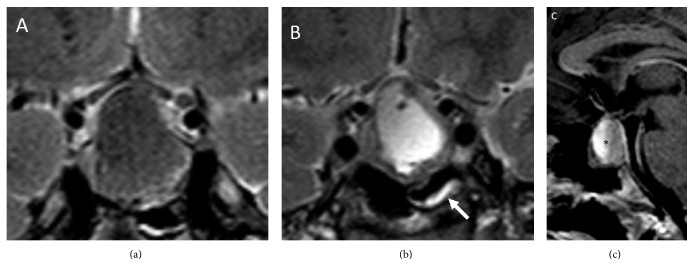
Coronal T2-weighted MRI images ((a) and (b)) and sagittal T1-weighted image (c) in patients with pituitary apoplexy showing (a) the low-signal haemorrhagic content of the pituitary mass, (b) the high-signal cystic area inside the lesion and the focal mucosal thickening of the sphenoid sinus (white arrow), and (c) a fluid-fluid intralesional level (asterisk) which is pathognomonic of pituitary apoplexy.
